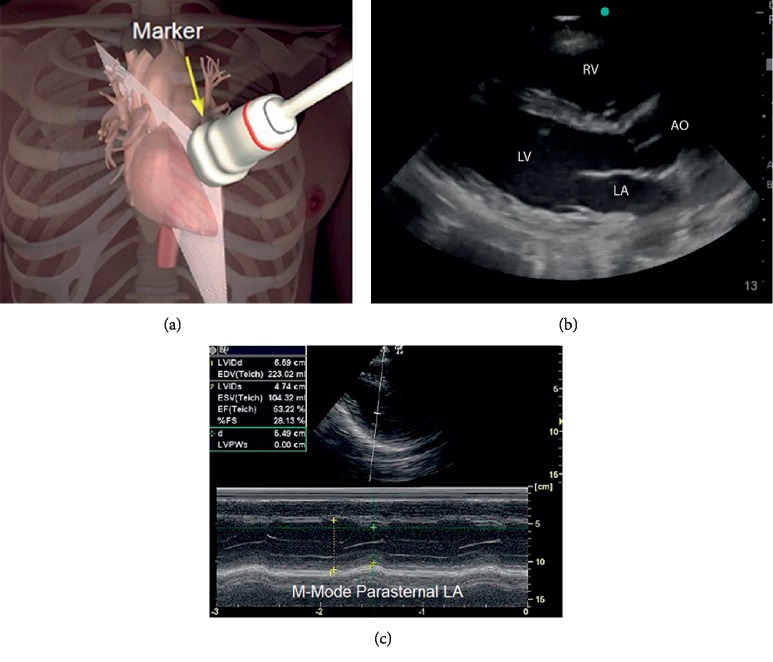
(a) Transducer position on the chest, left parasternal 4-5^th^ intercostal space. (b) Long axis view of the heart. (c) M-mode measuring the ejection fraction (EF).
